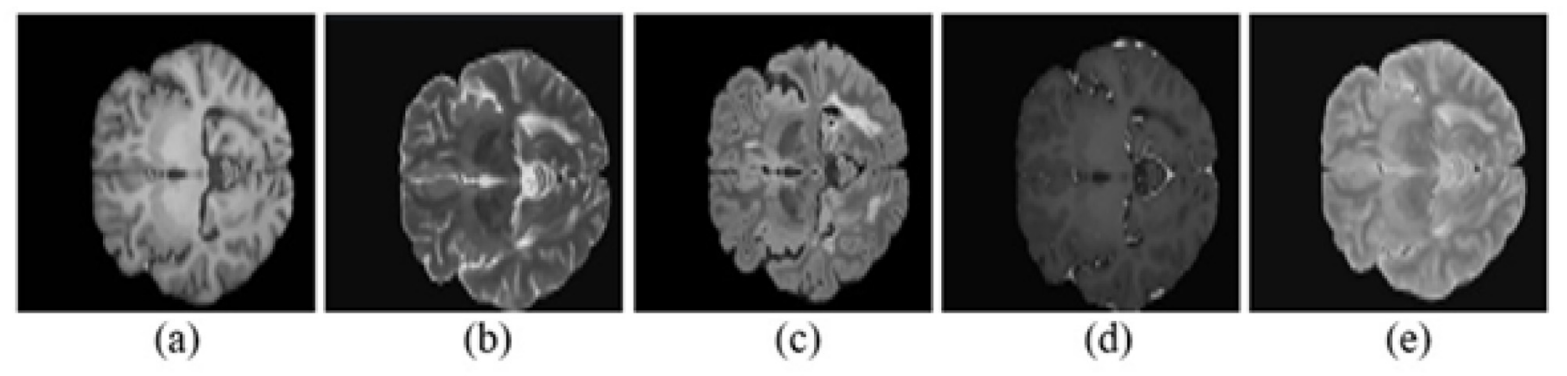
MICCAI-2016 dataset sample brain MRI in different image modalities when slice number is 282 for the third patient in the dataset. (a) T1-weighted. (b) T2-weighted. (c) FLAIR. (d) T1-Gd (gadolinium contrast). (e) DP-proton density.
